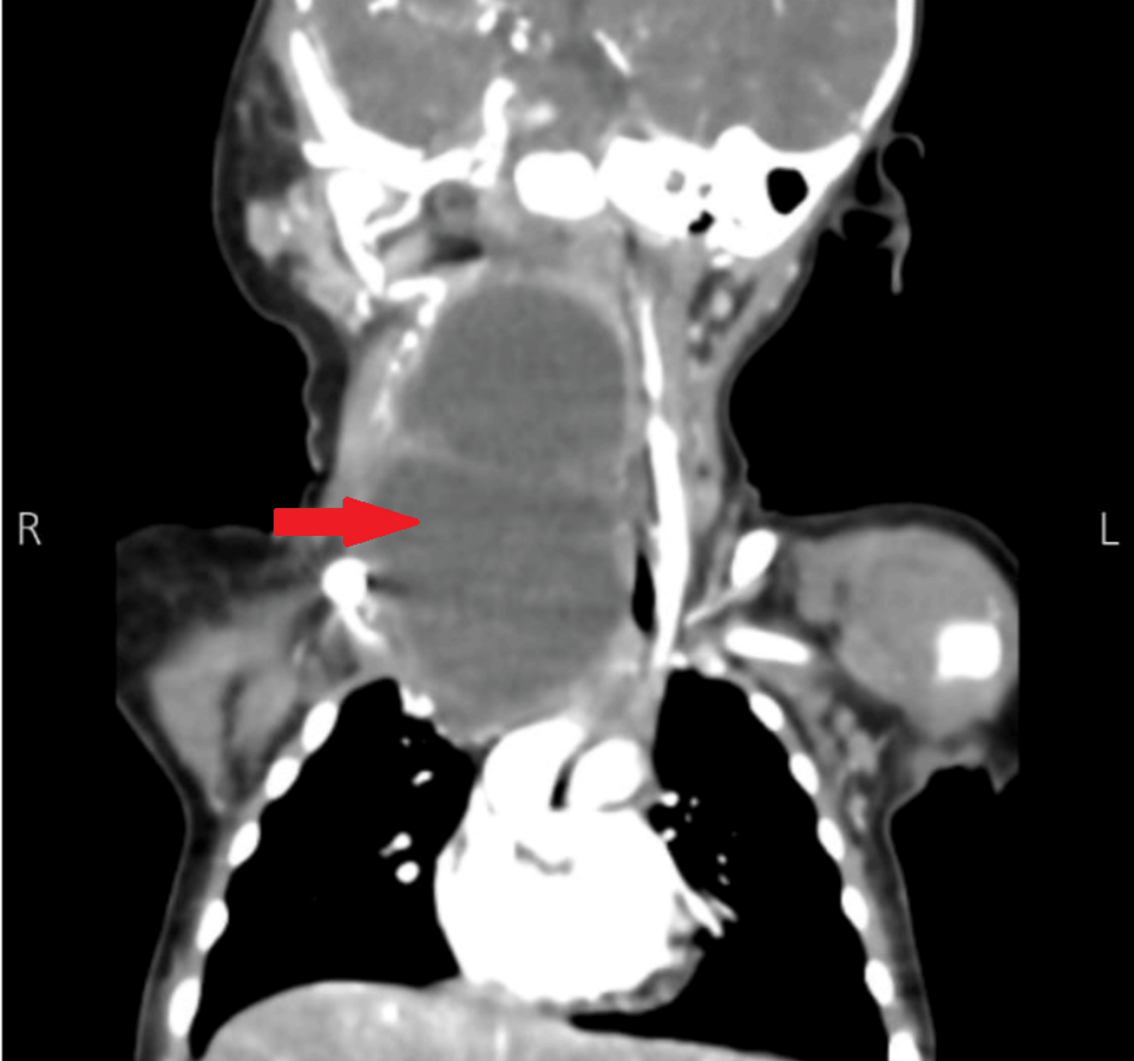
The coronal section of the contrast-enhanced CT scan shows 3.5×3.1×5.6 cm of cervical mass extending to the mediastinum, shifting cervical structures, and causing a mass effect (red arrow). CT: computed tomography
